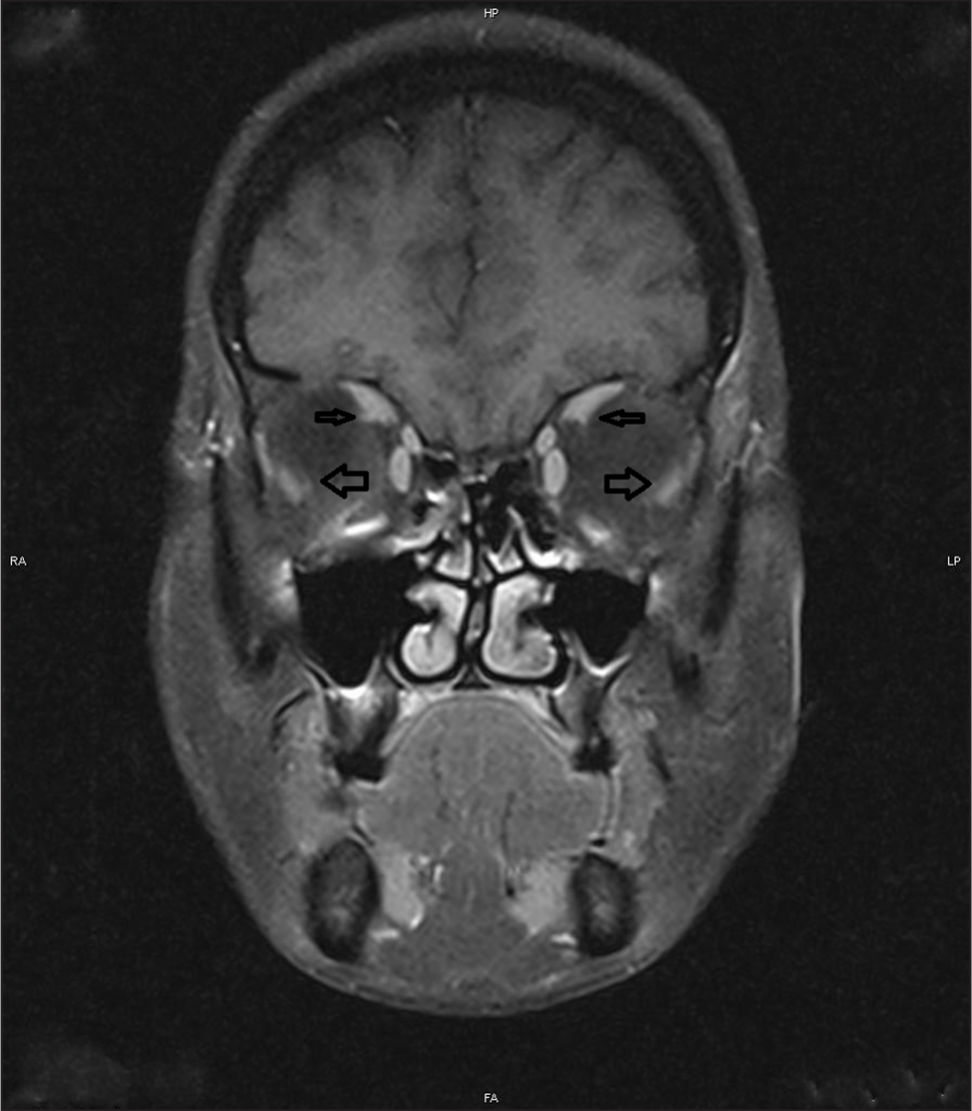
Magnetic resonance imaging of the orbit showing superotemporal herniation of the eyeball with inferior displaced lateral rectus (thick arrows) and nasally displaced superior rectus muscles (thin arrows).
